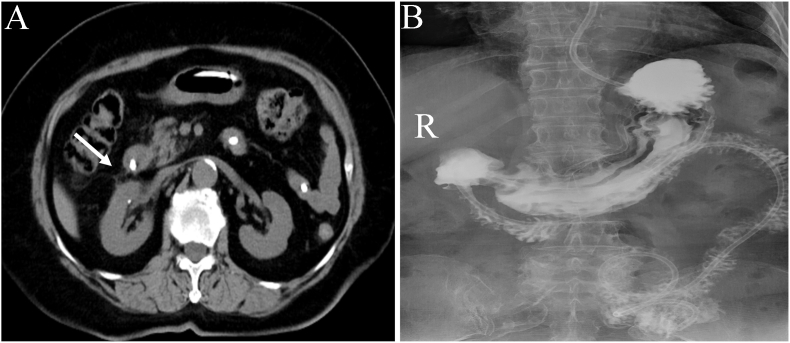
(A) A subsequent CT examination conducted one month later verified the complete resolution of the right renal cyst, as well as a clearly delineated boundary between the duodenum and the right renal periphery (white arrows). (B) The upper gastrointestinal series indicated no contrast extravasation from the duodenal bulb area one month later.
